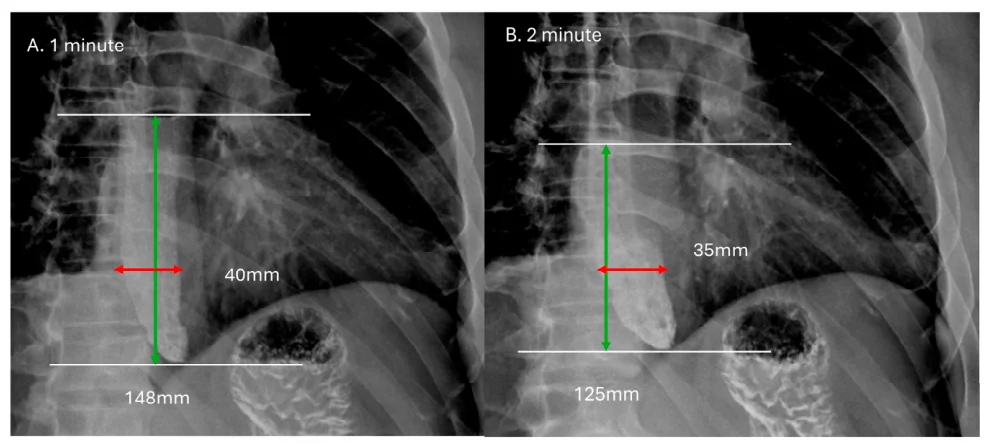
Representative timed barium esophagogram showing measurement of liquid barium retention over time. Left posterior oblique spot radiographs obtained at 1 min (A) and 2 min (B) after barium ingestion demonstrate persistent distal esophageal liquid barium retention. The vertical green line indicates barium column height, measured between the upper and lower limits of the retained contrast column, while the horizontal red line indicates maximal column width. In this example, column height decreased from 148 mm at 1 min to 125 mm at 2 min, and maximal width decreased from 40 mm to 35 mm.
